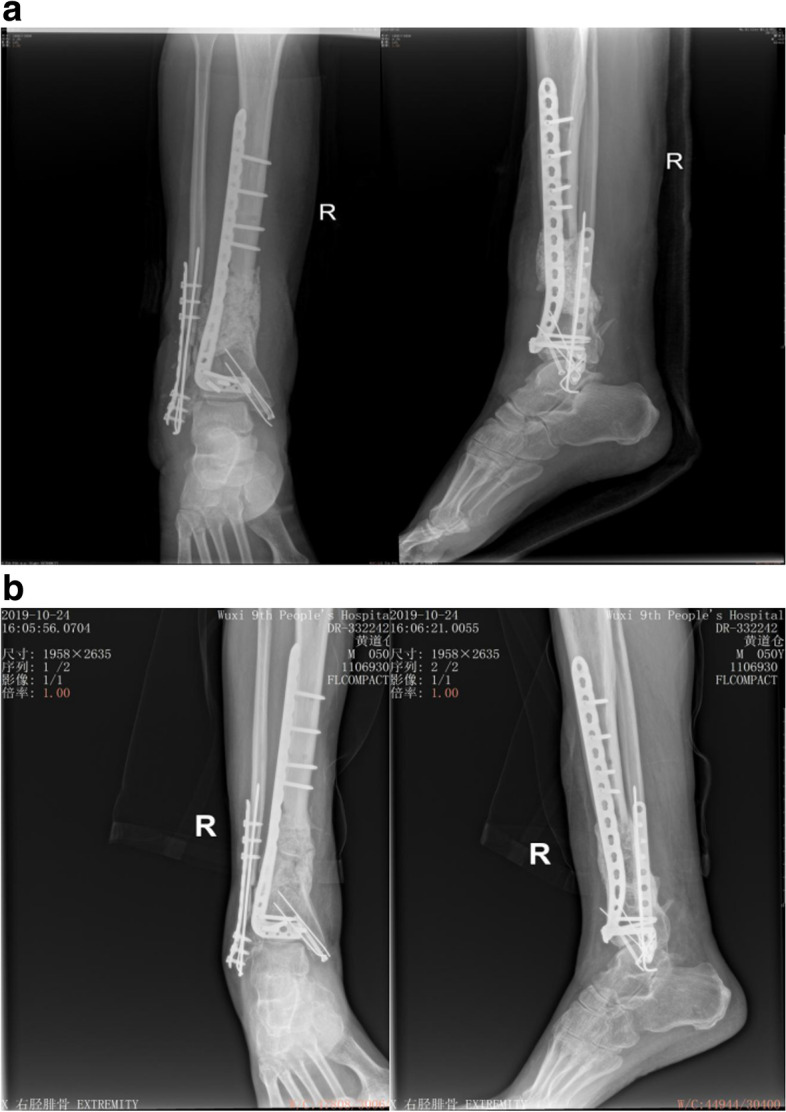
A 47 years-old male patient with segmental defects of the right tibia treated with IMT. a The cement spacer was prepared by intracorporeal formation. b X-rays showed the defects clinically healed in 7 months
